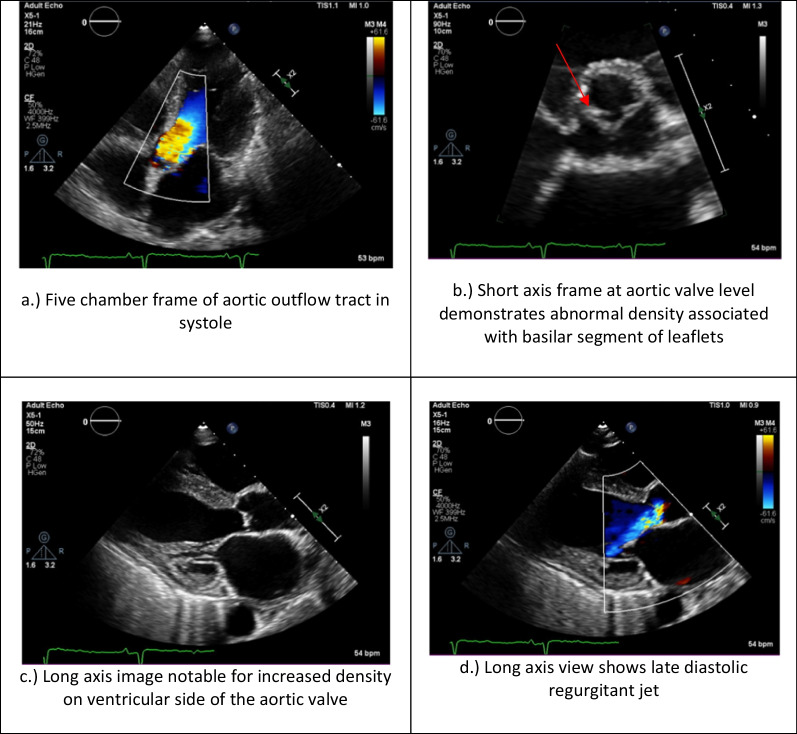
Transthoracic echocardiogram series
